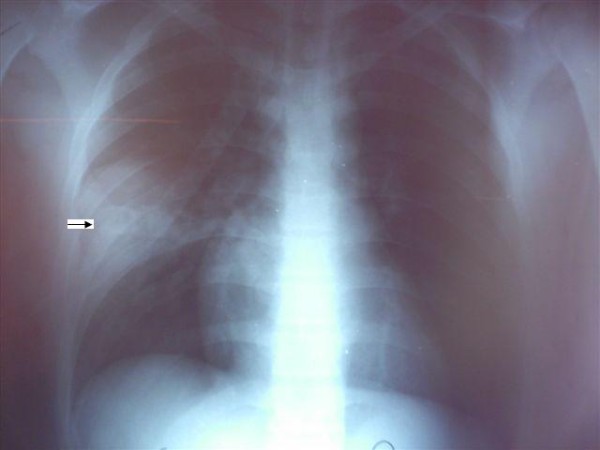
Admission chest radiography. Admission chest radiograph shows a radiopaque image within a pulmonary contusion (arrow), and a small pneumothorax on the right hemithorax.
